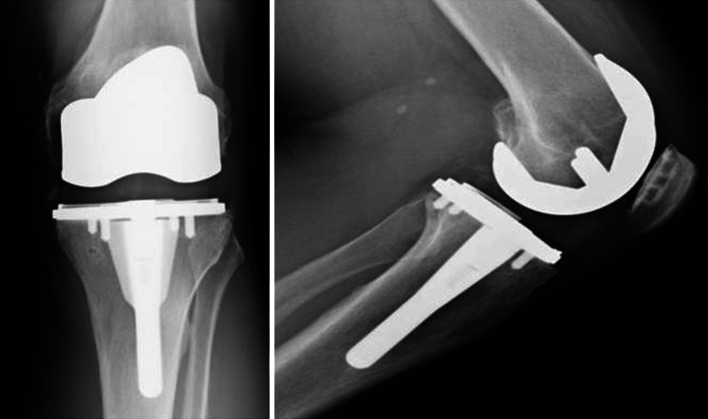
Representative anterior–posterior and lateral radiographs of a well-fixed BIOFOAM-coated tibial component
